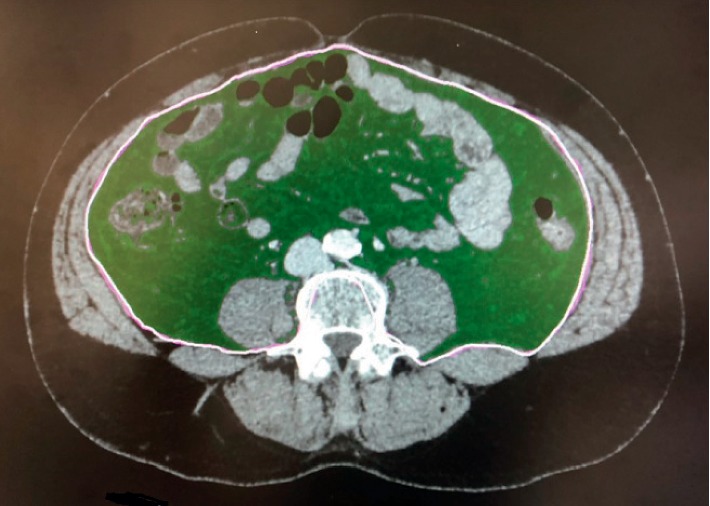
Area of visceral fat at the level of the umbilicus (green field) measured on preoperative computed tomography with AquariusNET server.
